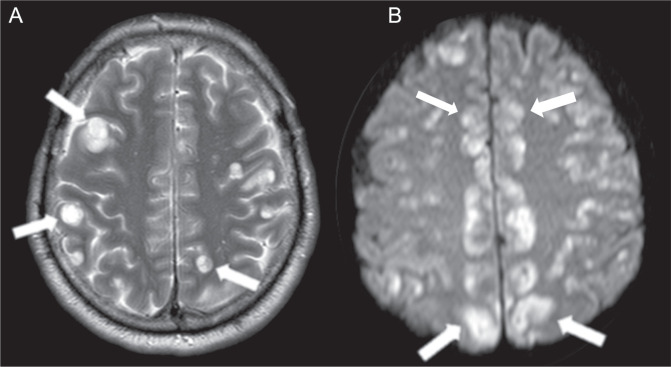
MRI scans of two different patients. A: Axial T2WI demonstrating
